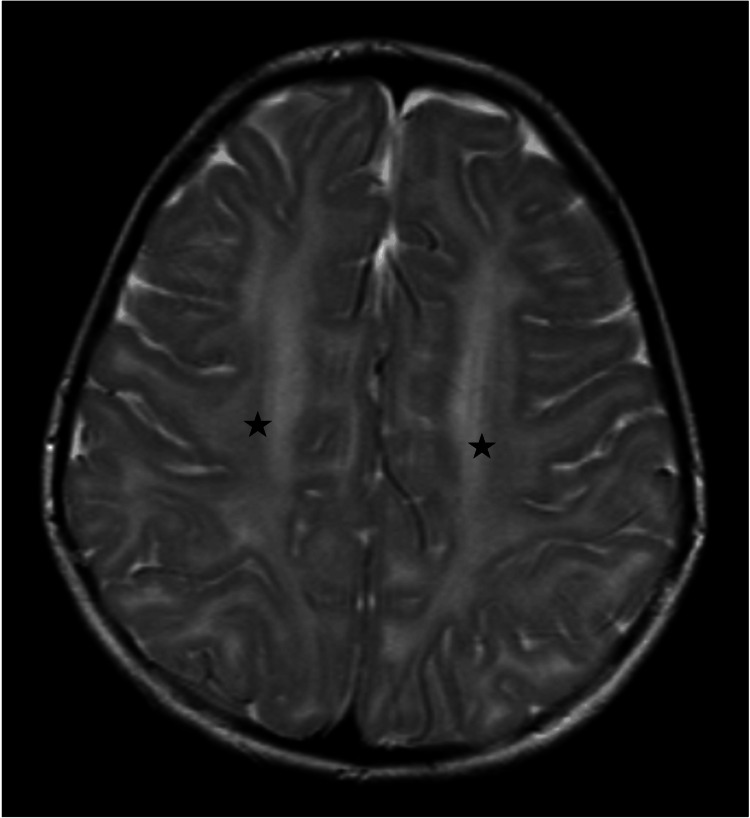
Axial T2 Weighted Imaging Axial T2-weighted image at the level of centrum semiovale obtained at 23 months of age demonstrated extensive, confluent, symmetric T2 hyperintense signal (asterix) involving the periventricular, deep, and subcortical white matter attributed to hypomyelination and metabolite accumulation.
